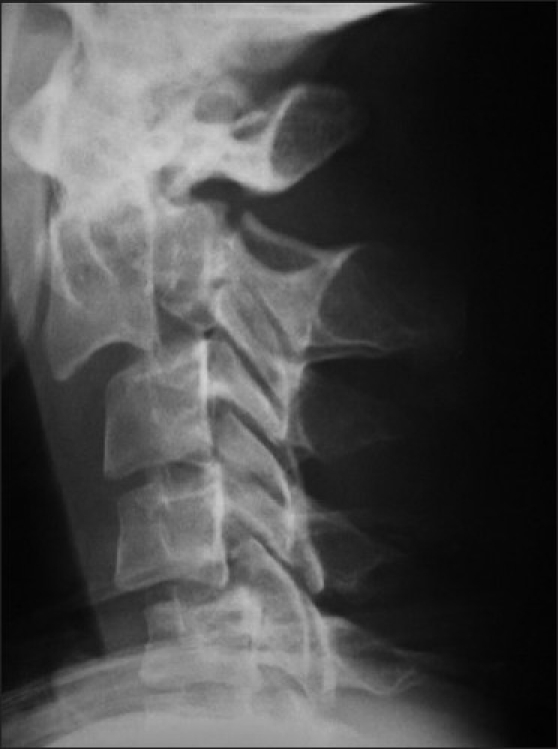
Radiography showed dens fracture on C2 (type III hangman’s fracture)
